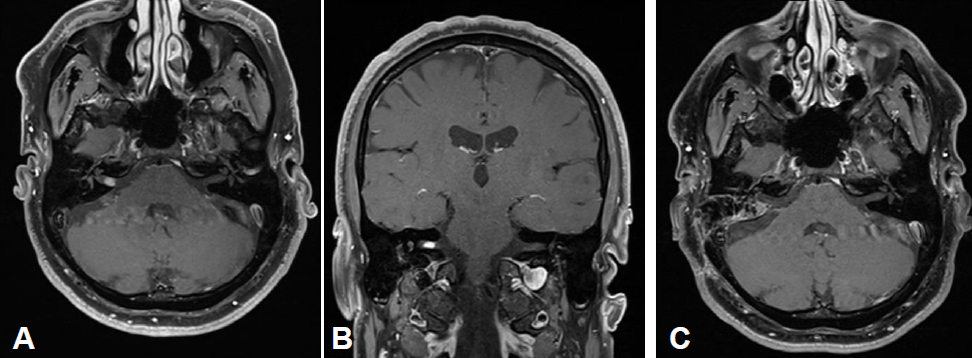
Preoperative MRI, T1 + CM; A axial; B coronal; 6x4x3 mm measuring intrameatal vestibular schwannoma on the right side. MRT, T1 + CM axial; C 5 months after surgery no hint for residual tumor or recurrent after translabyrinthine resection.
